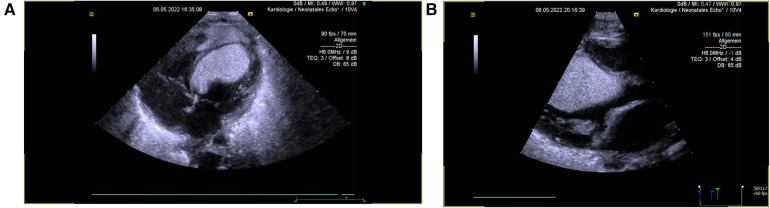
Echocardiography 2 h after birth: apical four-chamber view (A) and parasternal long axis (B) showing one very large tumor in the LV cavity, originating from the septum (30.5 × 18 mm^2^).
